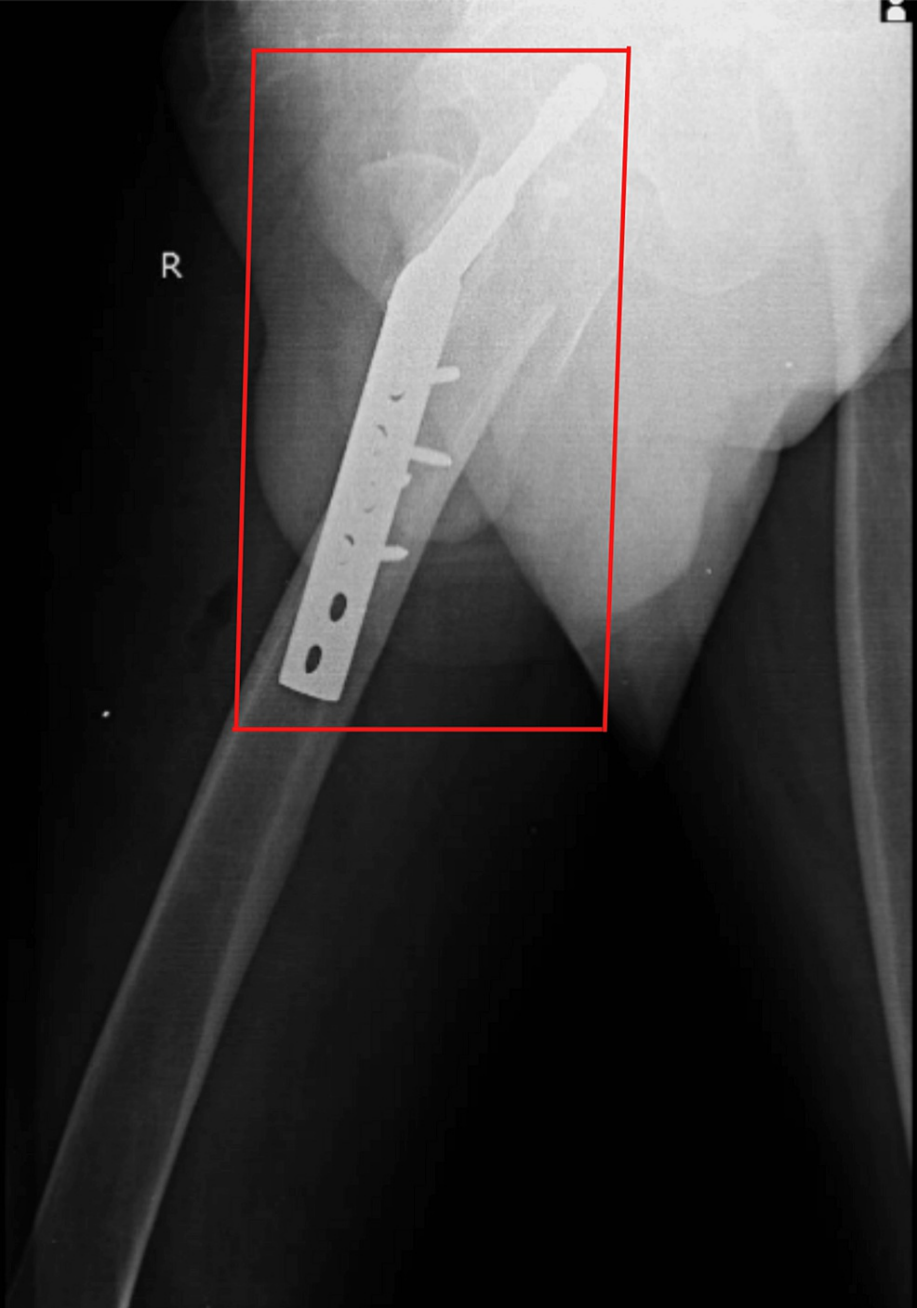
Displays a lateral view of an X-ray showing dynamic hip screw fixation of fracture fragments.
